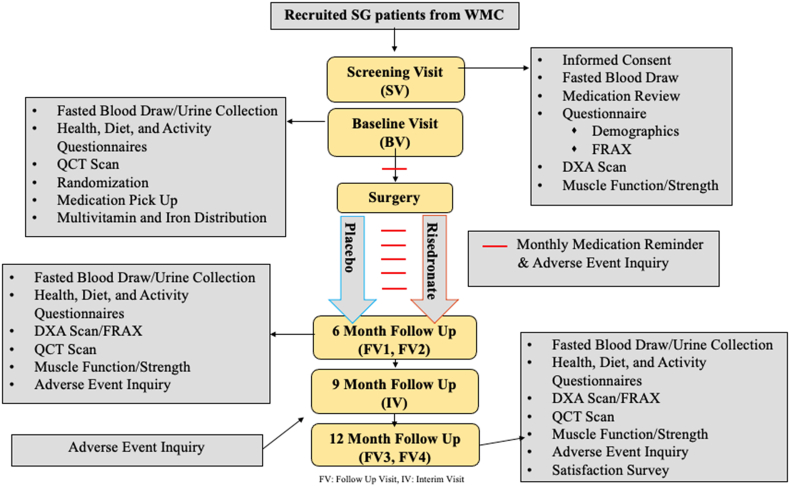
Outline of study flow. FRAX: Fracture Risk Assessment Tool; DXA: Dual-Energy X-Ray Absorptiometry; QCT: Quantitative Computed Tomography; FV: Follow Up Visit; IV: Interim Visit.
